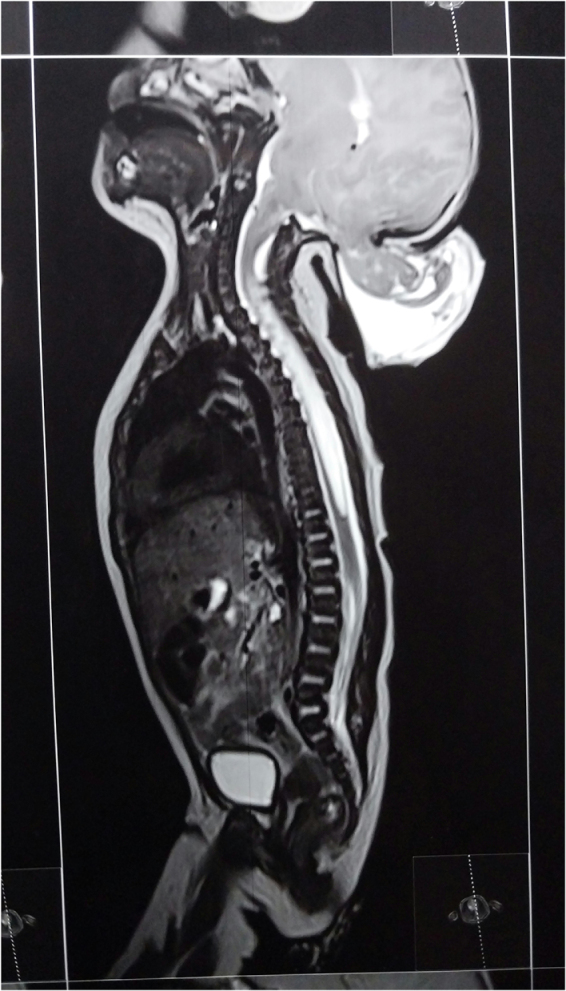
T2-weighted MRI showing a syrinx extending from C5–C6 to L1–L2 with mild cord expansion with maximum diameter is 8.1 mm and syrinx-to-cord ratio of 8.1:13.
